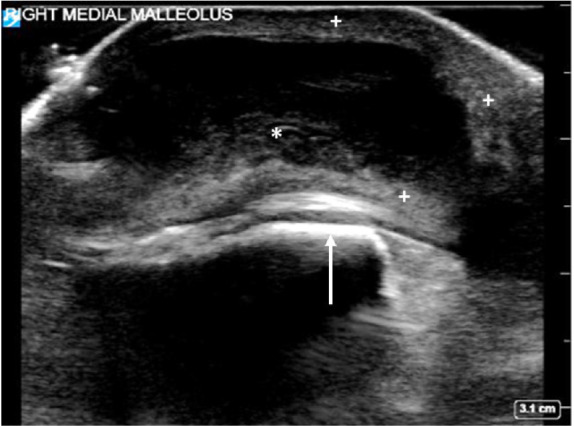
Ultrasound demonstrating a thick-walled (plus signs) bursa containing complex fluid (asterisk), which overlies the medial malleolus (arrow).
